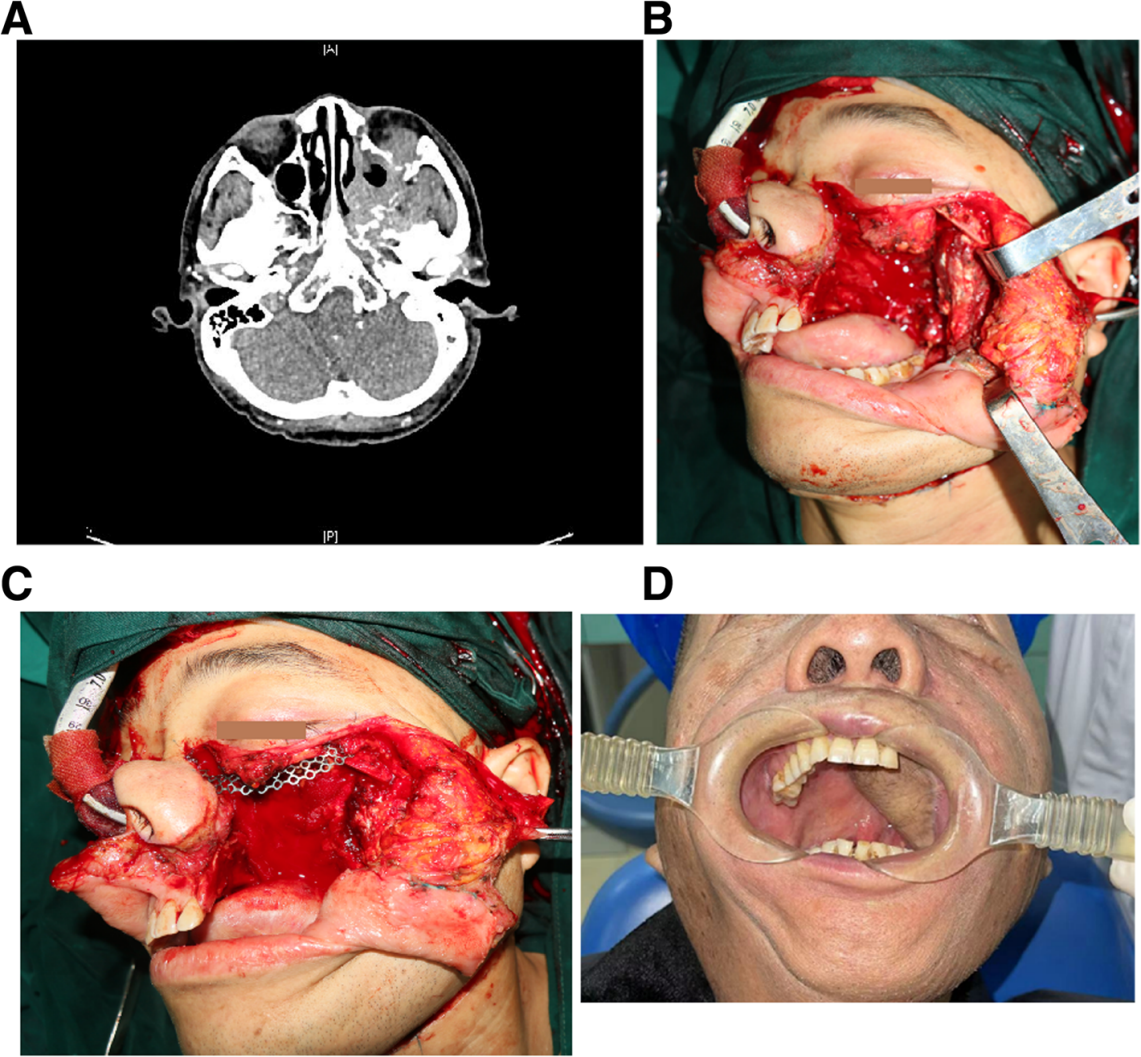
A 51-year-old man with stage IV palatal ACC underwent total maxillectomy and ipsilateral selective neck dissection. Computed tomography scan showing that the tumor had broken through the inferior orbital wall and entered the orbit (A). Incision of the tumor and defect (B). Reconstruction of the inferior orbital wall and class III maxillary defects with titanium mesh (C) and a free anterolateral thigh flap (D)
